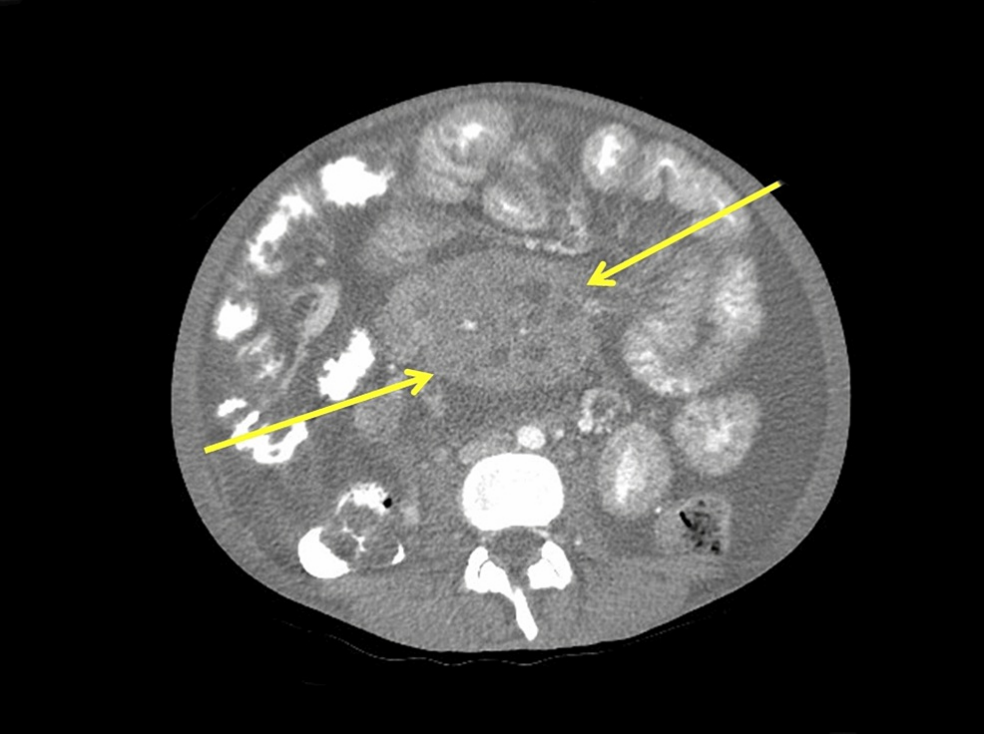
Abdominal CT showing mesenteric lymphadenopathy without splenomegaly
